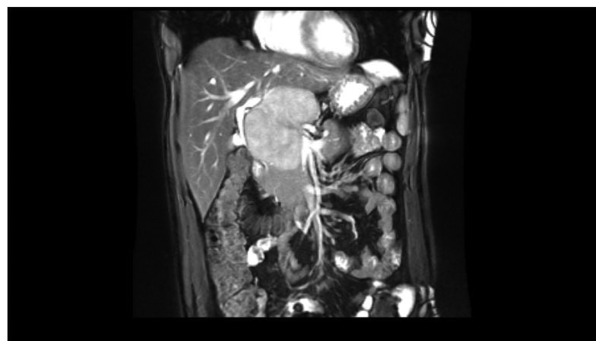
Coronal T2-weighted fat-suppressed magnetic resonance image shows a marked hyperintense pancreatic head mass.
